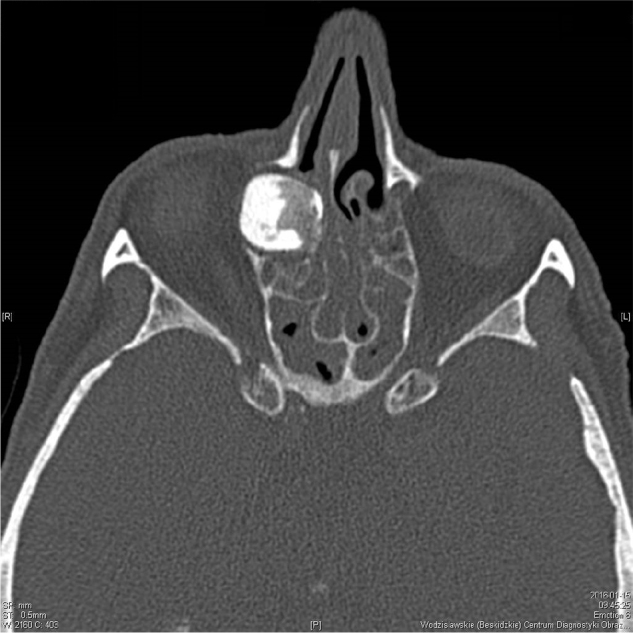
CT scan of the sinuses – fronto-ethmoidal osteoma, axial plane.
